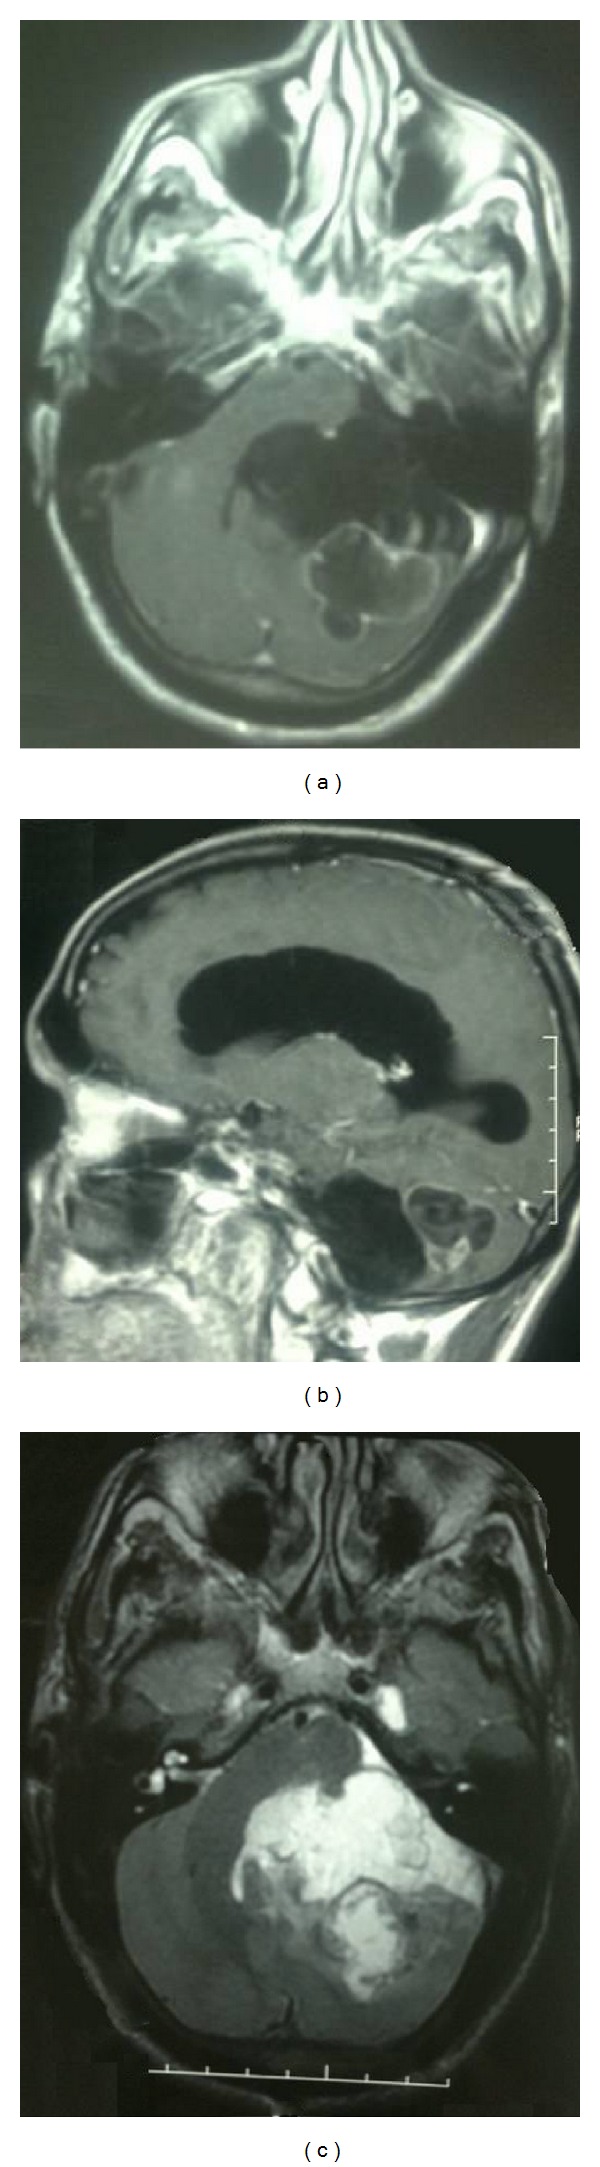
Axial (a) and sagittal (b) T1-weighted MRI with gadolinium and axial (c) T2-weighted MRI before surgery revealing a large cystic lesion of left CPA with enhancement of lower portion of the lesion and severe compression of forth ventricle and brainstem.
